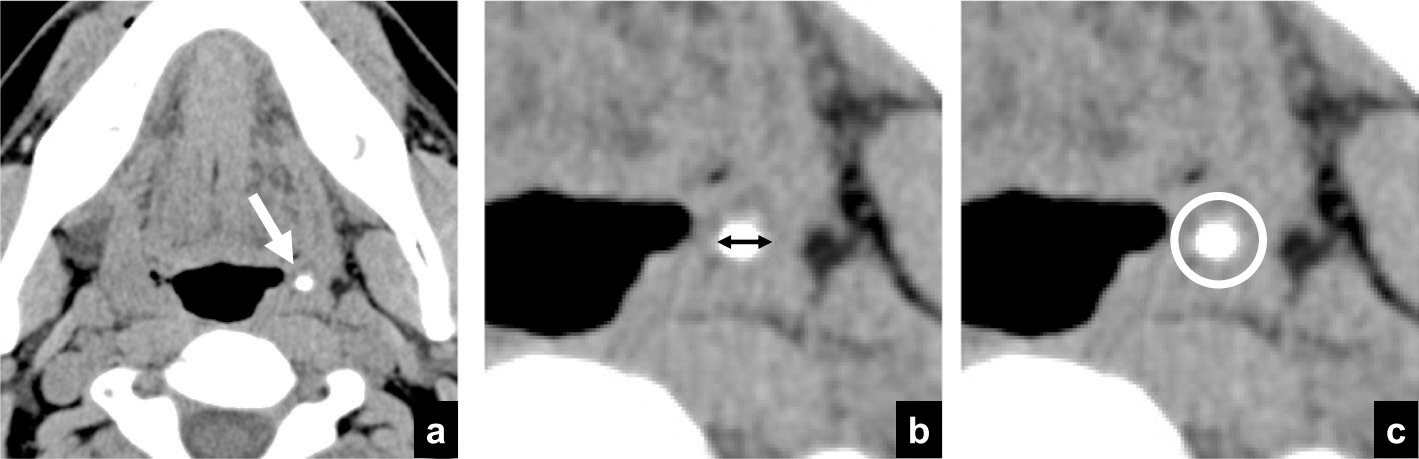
Methods for measurement of sizes and CT values of tonsilloliths on CT images. a The largest calculus (soft-tissue window setting, arrow) in the palatine tonsil is included in the analysis. b Size is defined as the long axis measurement (arrow) on the soft-tissue window setting. c The calcification level is defined as the maximum CT value on the soft-tissue window setting, and the region of interest includes the whole calculus (circle)
